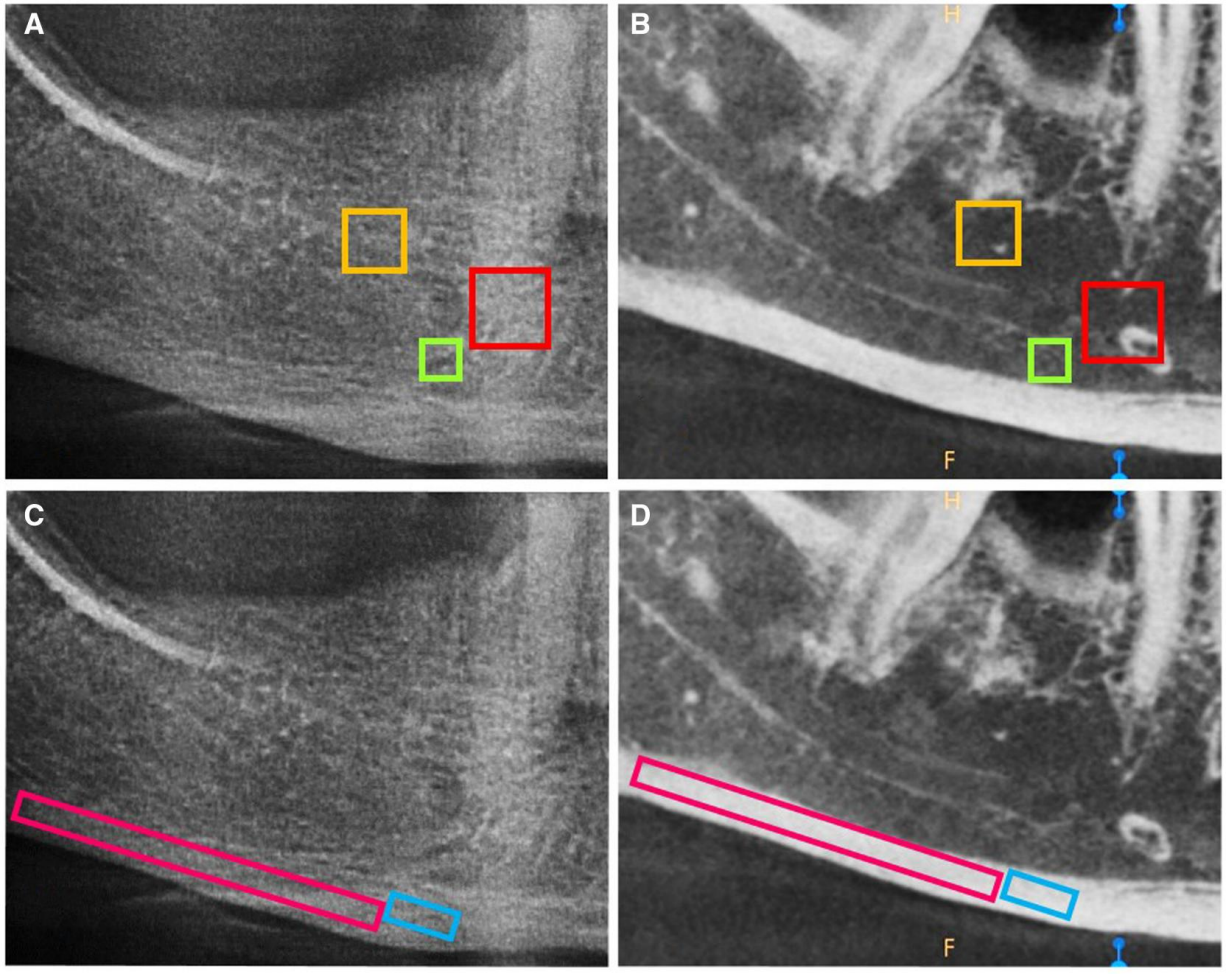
Locations of trabecular and cortical ROIs in PR and sagittal CBCT images. (A) PR and (B) CBCT images showing trabecular ROIs anterior to the mental foramen (ROI-1), inferior to the mental foramen (ROI-2), and distal to the mental foramen above the inferior alveolar canal (ROI-3). (C) PR and (D) CBCT images showing cortical ROIs inferior to the mental foramen (ROI-4), and between the distal border of the mental foramen to the ascending ramus (ROI-5).
